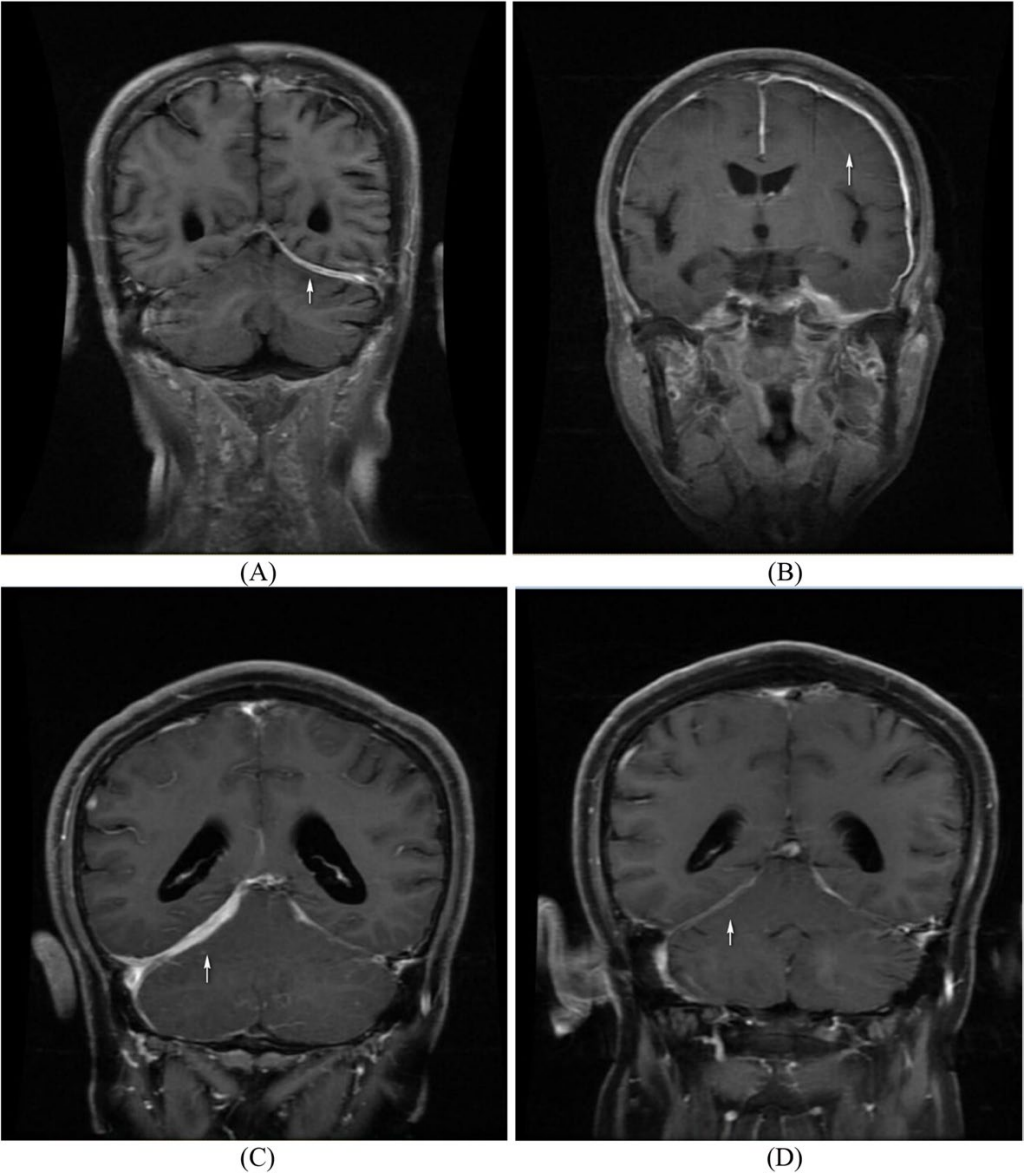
Brain magnetic resonance imaging of patients with GPA and HCP. The dural thickness of the left tentorium cerebella and left frontotemporal lobe are indicated by the arrows in (A) and (B) on T1-weighted gadolinium-enhanced images. The changes of tentorium cerebelli before and after treatment in the same patient are shown in (C) and (D). GPA, granulomatosis with polyangiitis; HCP, hypertrophic cranial pachymeningitis
